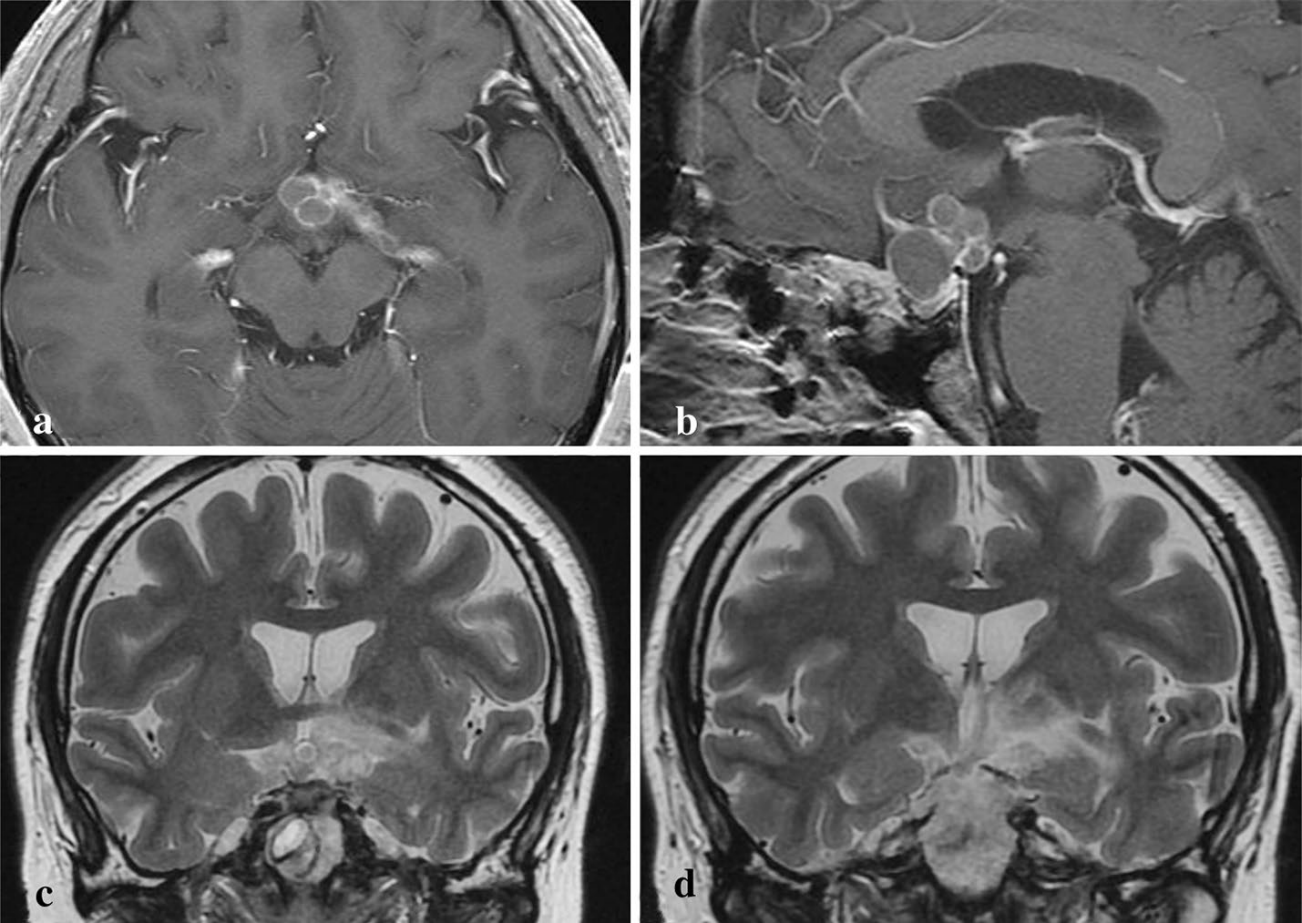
Case 1. a Preoperative axial T1-weighted MR image with gadolinium revealing a multicystic sellar lesion extending from the suprasellar cistern to the left ambient cistern. b Preoperative sagittal T1-weighted MR image with gadolinium revealing infiltration of the lesion to the third ventricle floor. (c, d) Preoperative coronal T2-weighted MR images revealing an extensive irregular high intensity area in the left hypothalamus
